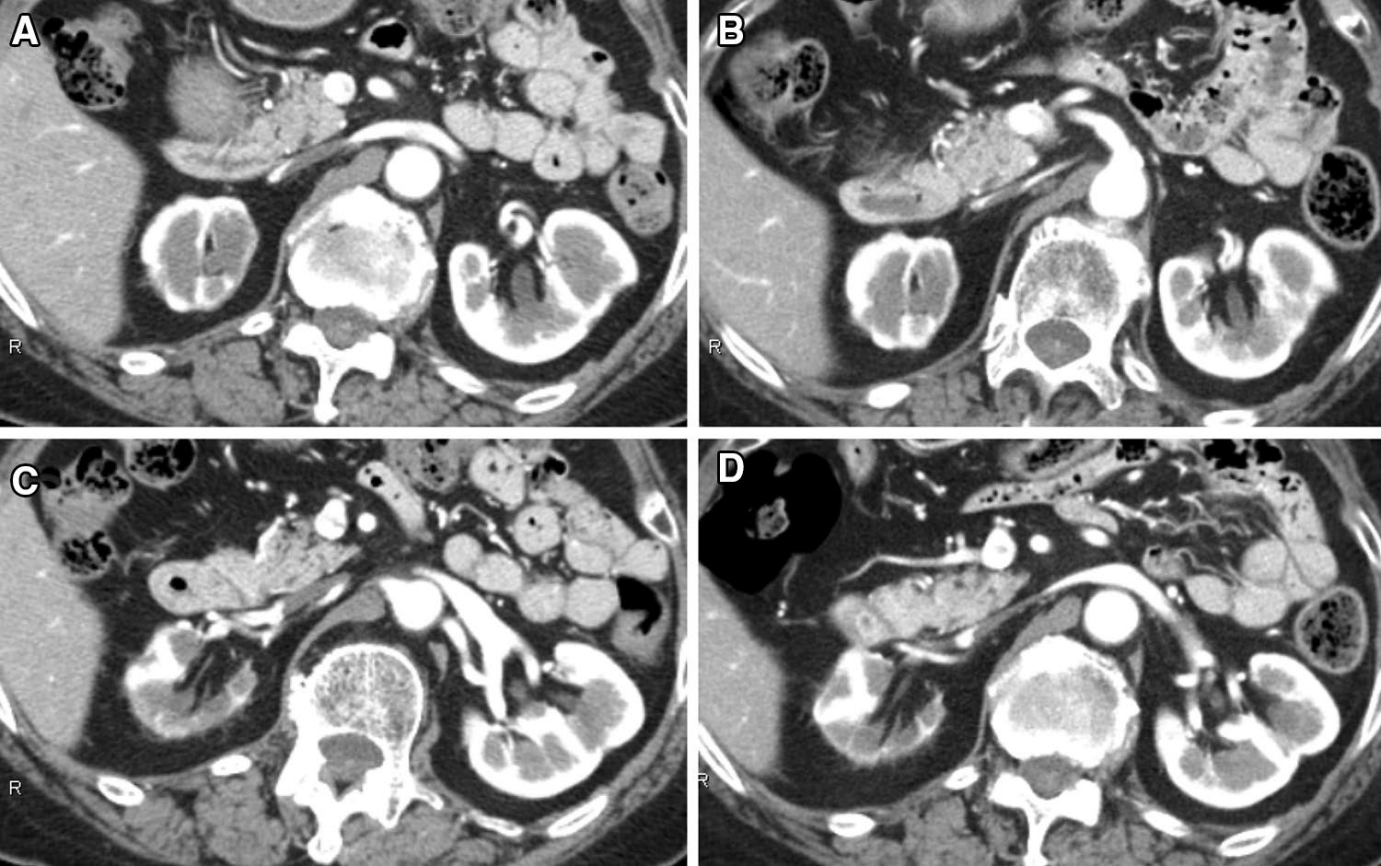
Contrast-enhanced computed tomography images on admission (a, c) and 1 month after glucocorticoid therapy (b, d). After steroid therapy, the left kidney lesions showing low density and mild partial atrophy demonstrated almost no change
